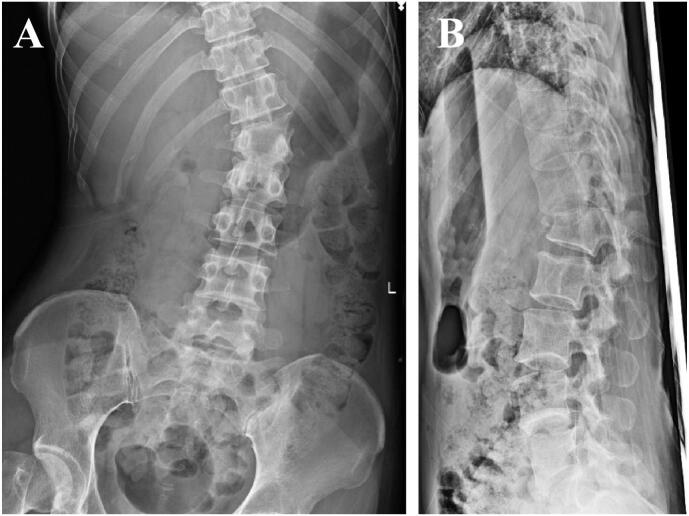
Simple roentgenograms revealed a dislocated fracture of Th12/L1 and fractures of the right 10th–12th ribs (A, B).
